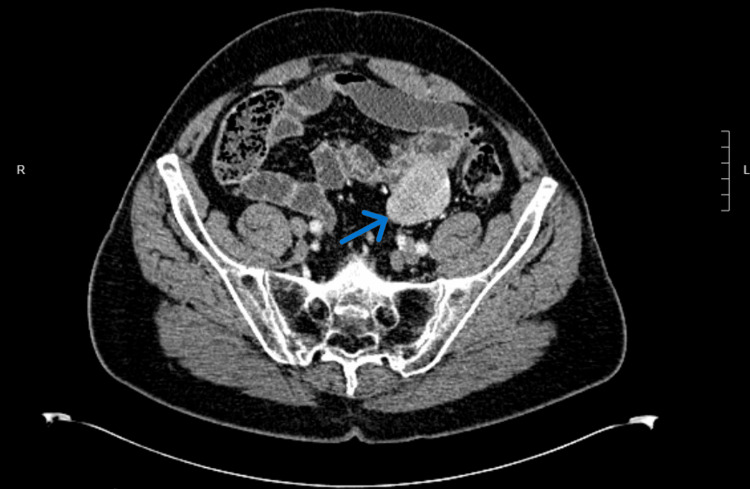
CT enterography three years after hiatal hernia repair showing a small bowel mass.
